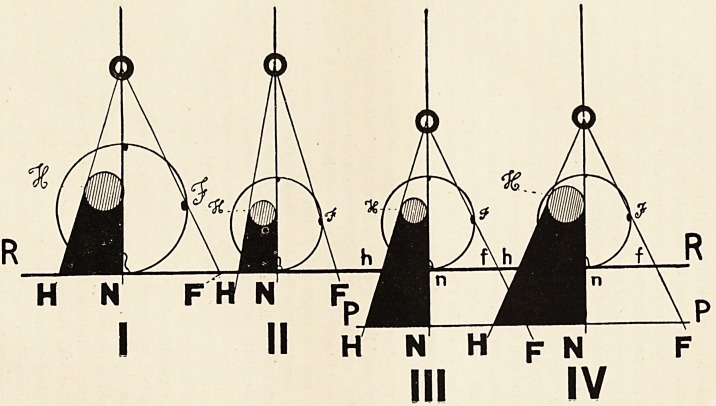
# Proportional Representation and the Comparison of Radiographs
